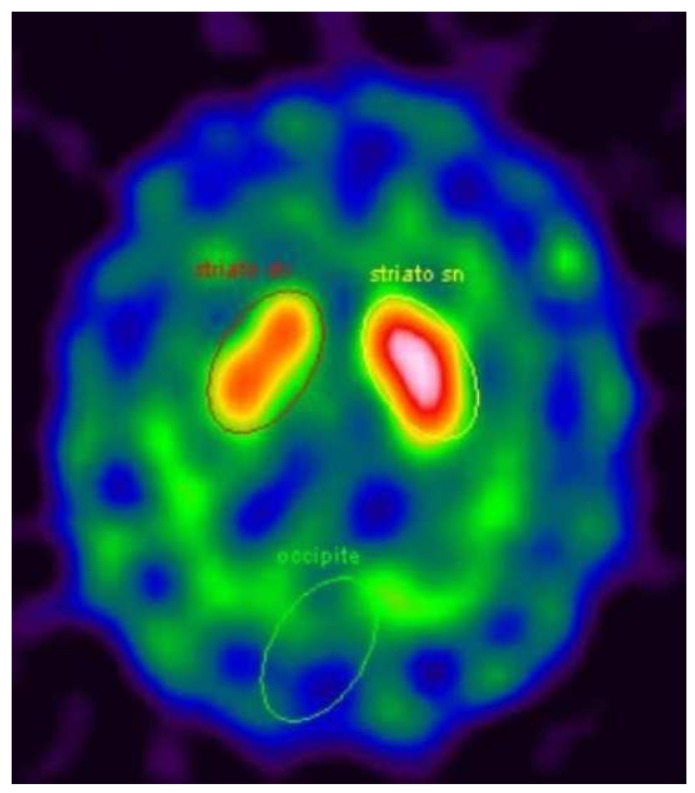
DaT-Scan SPECT showing asymmetric reduction of dopamine transporters in right striatum.
